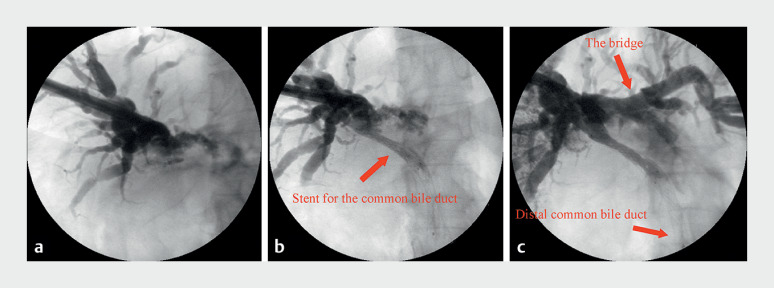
X-ray examination with contrast agent injected through the sinus.
